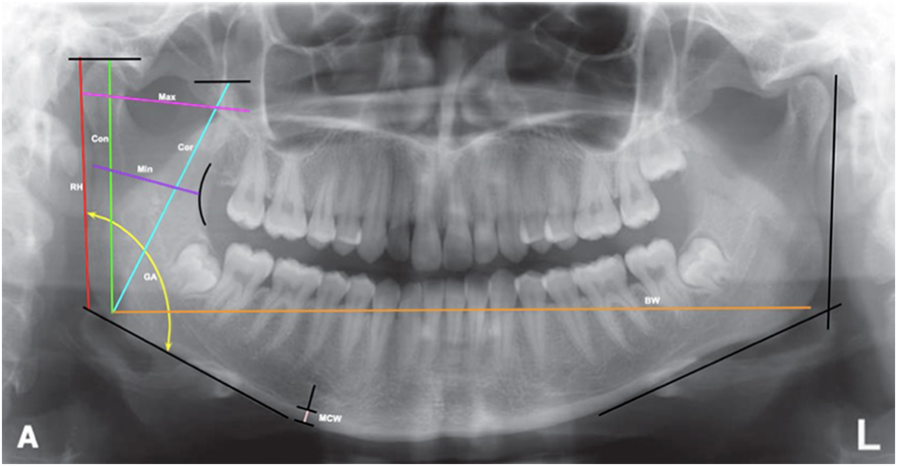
Measurement of mandibular parameters [ramus height (RH), maximum ramus width (max), minimum ramus width (min), gonial angle (GA), bigonial width (BW), condylar process height (Con), coronoid process height (Cor), and mandibular cortical width (MCW)] on panoramic radiographs.
